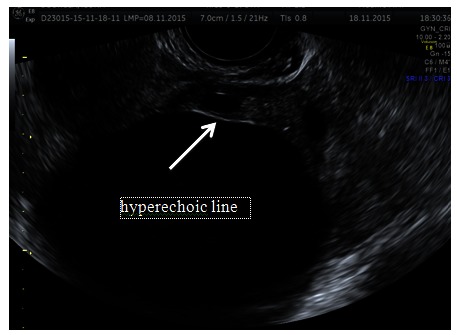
Conventional 2D transvaginal ultrasound evaluation of a paraovarian cyst; the hyperechoic line is always visible between the cyst and ovarian capsule when the cyst does not originate from the ovarian tissue
